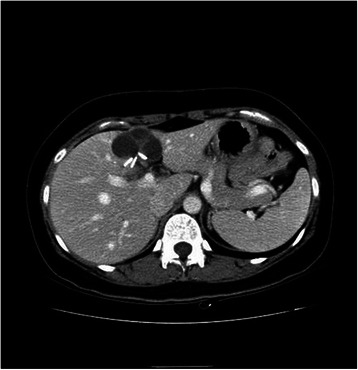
Postoperative recurrence after liver-preserving surgery. A 38 year-old female received laparoscopic cystic fenestration for intrahepatic biliary cystadenoma. Four months after the operation, tumor recurrence was documented by CT. The high density objects beside the cystic tumor were hemoclips used to secure blood vessels
